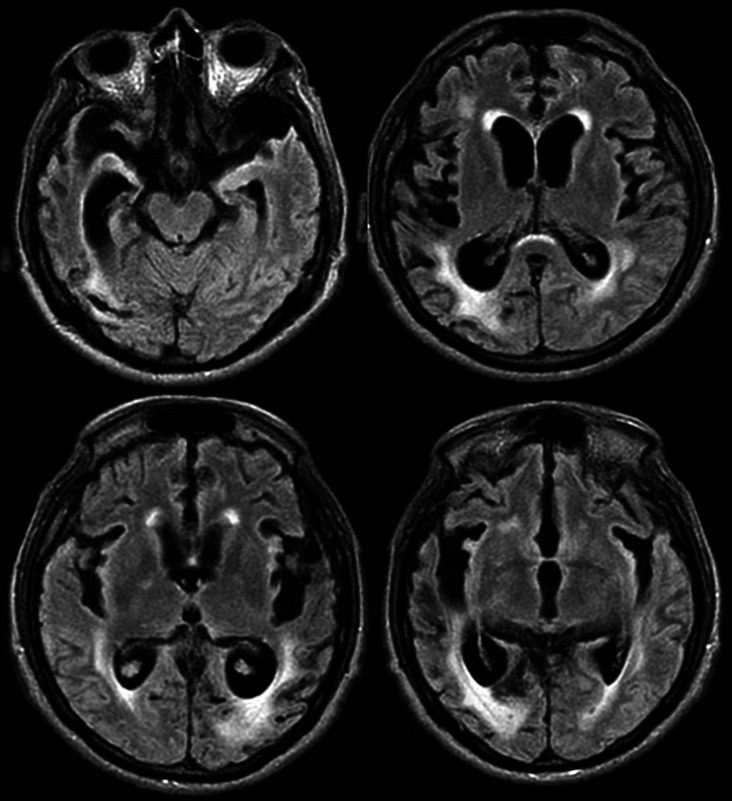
Axial brain-MRI of the patient with probable sporadic CJD. Axial section in a fluid attenuated inversion recovery (FLAIR) sequence showing hippocampal volume decreased in both temporal lobes with increase of temporal horns predominantly on right side; mild hyperintensity in right caudate nucleus and moderate in periventricular regions with confluent zones in the right parietal region; and moderate to severe atrophic cortical-subcortical with frontotemporal predominance.
